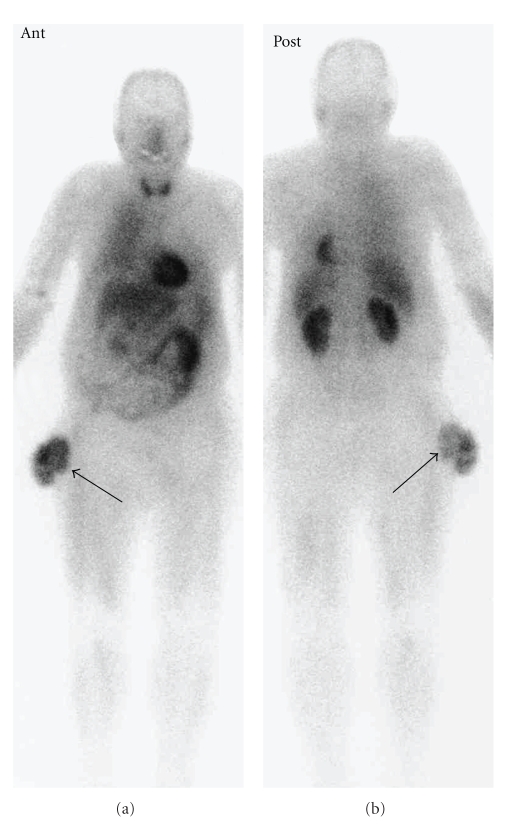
Thallium-201 scintigram of whole body in early phase. Abnormal accumulations accorded for the mass were detected (black arrows).
